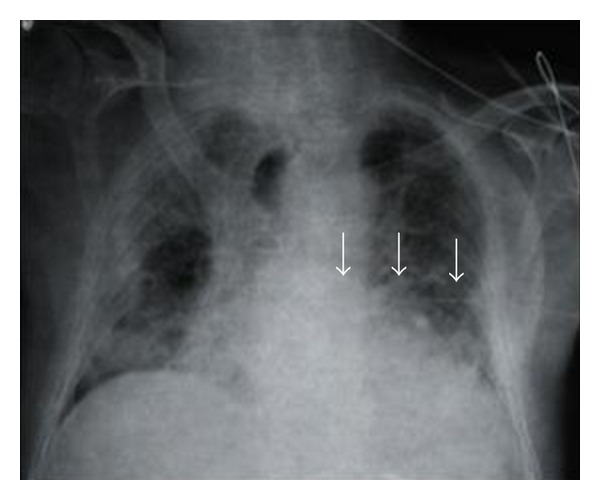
Chest radiography showing the standard aberrant course of the catheter left in the middle of the thorax (arrows).
